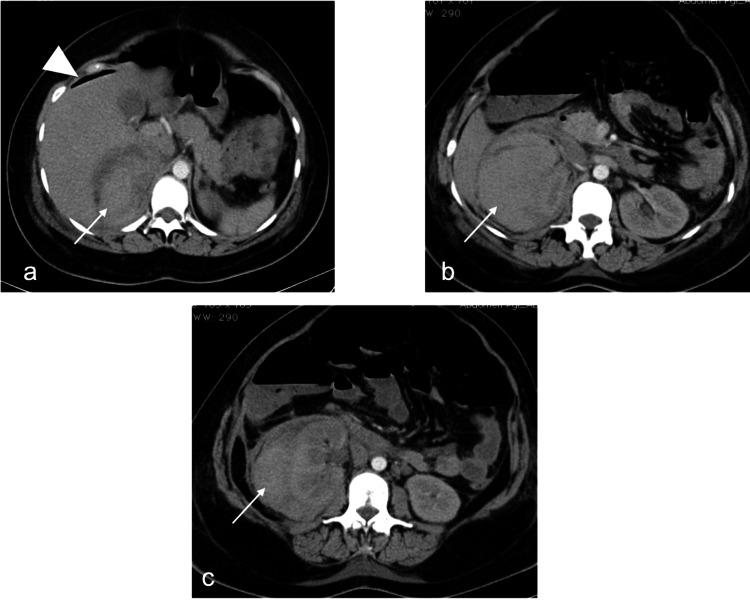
CT angiography findings. (a-c) Axial CT angiography images at the suprarenal and renal hilum level show heterogeneous enhancement with predominant hyperdense contents (arrow) in the right suprarenal space extending inferiorly into the perinephric space, suggesting right adrenal hemorrhage with hematoma. The right adrenal gland is not separately identified from the hematoma. A note is made of the pneumoperitoneum suggestive of the postoperative status (arrowhead in a).
